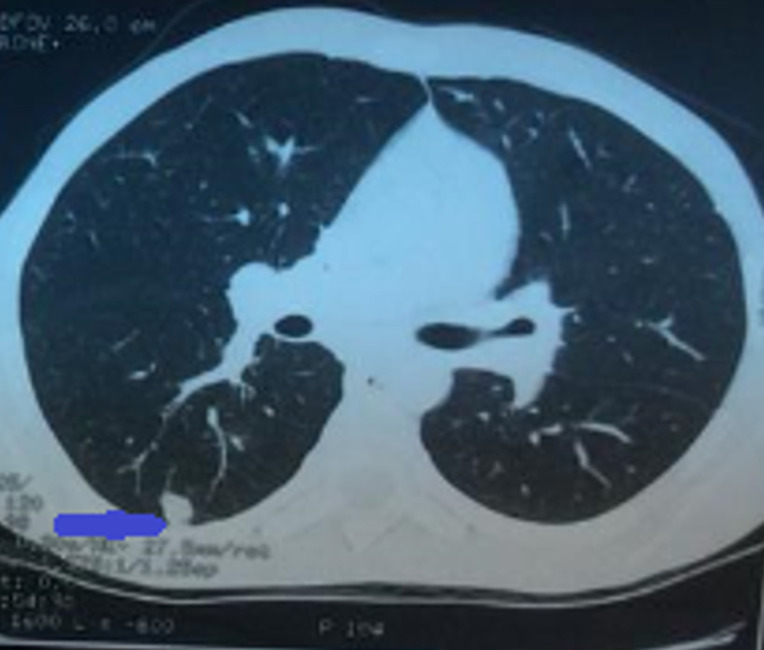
transversal (axial) CT scan of the chest showing condensation in the apical segment of the inferior right lobe
